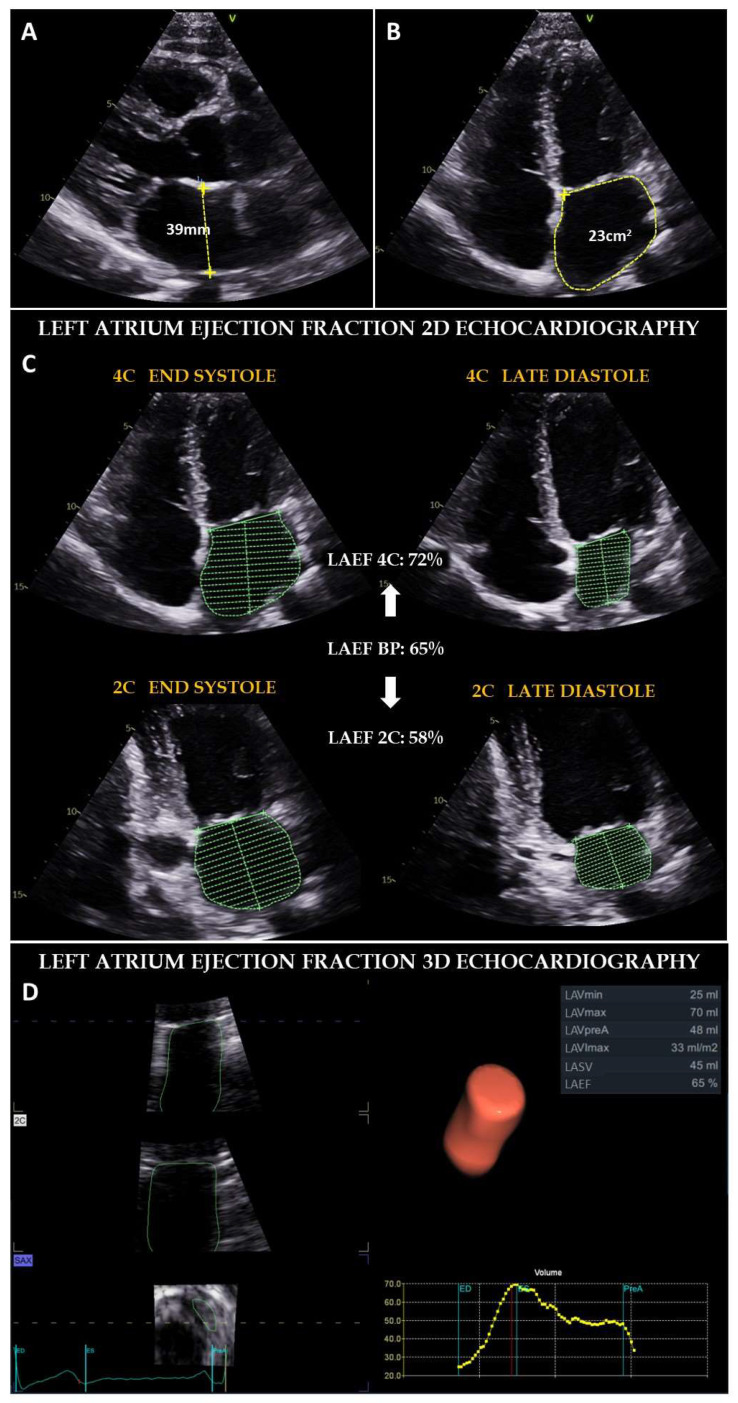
Methods for the determination of LA size and function by performing TTE. (A) Maximal anteroposterior LA diameter measured in 2 dimensions in parasternal long axis view from leading edge to leading edge, perpendicular to the posterior wall of LA. (B) Maximal LA area measured in apical four chamber view. (C) Total LA ejection fraction calculated by 2 dimensions in four and two chamber apical view. Maximum volume is assessed in end systole just before mitral valve opening. Minimum volume is estimated in late diastole in the onset of the P wave. (D) Total LA ejection fraction measured in 3 dimensions, acquiring a complete volume including the LA and applying specific software for the calculation.
